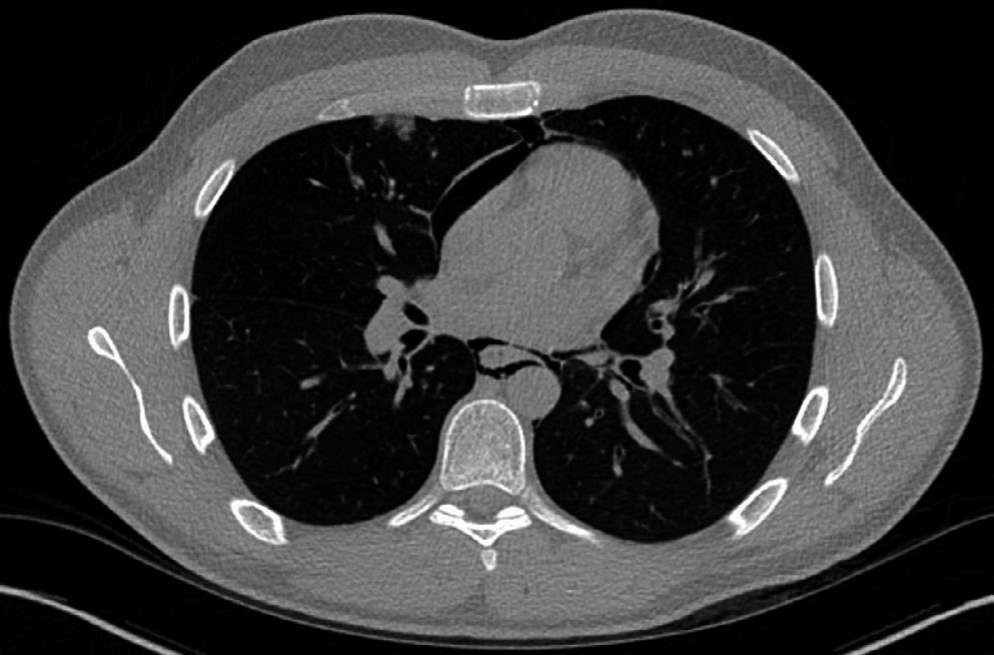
CT scan demonstrating pneumopericardium
